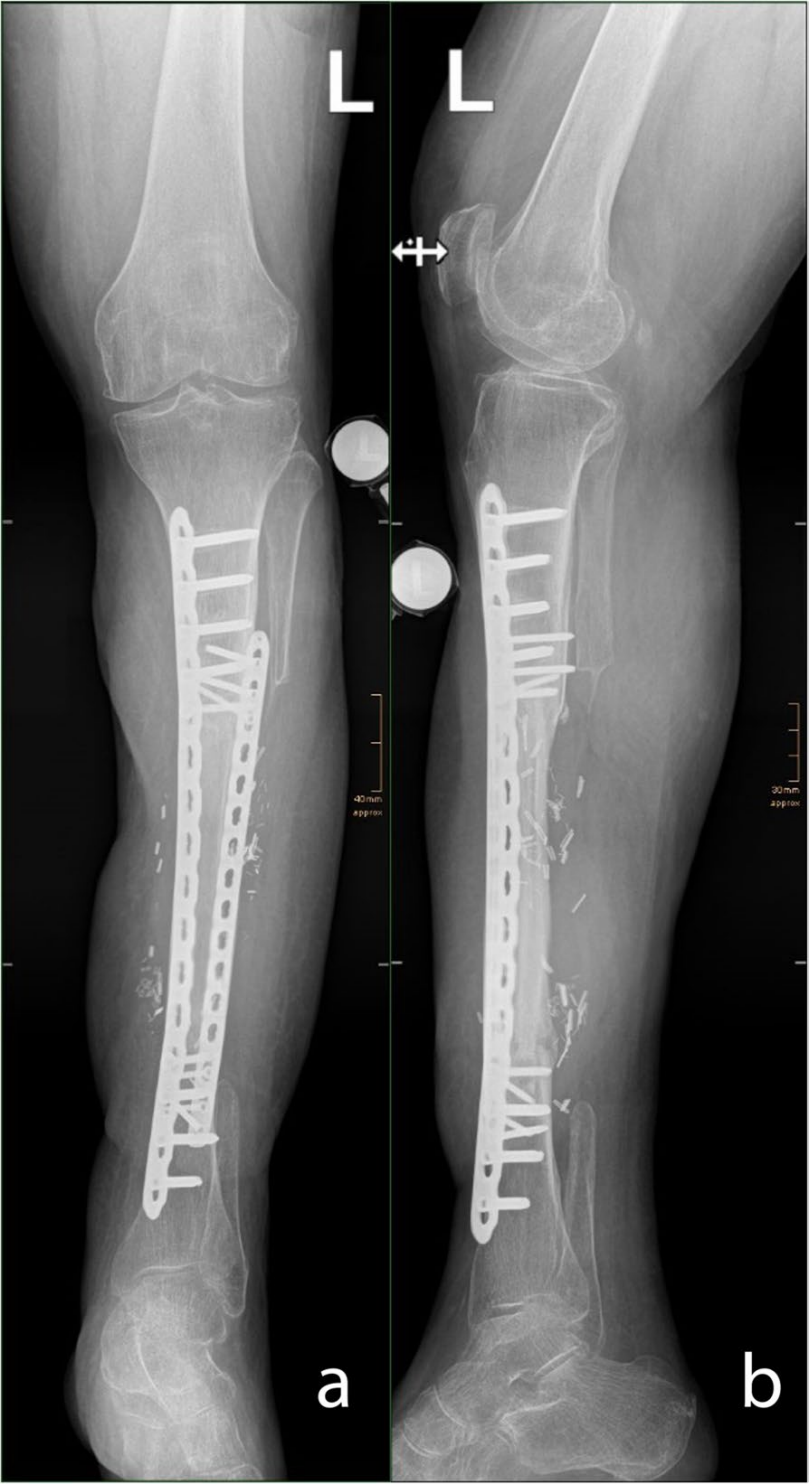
X-rays 2 years after the first surgery with the two plates and the fibular hypertrophy. a Anterior posterior view. b Lateral view
